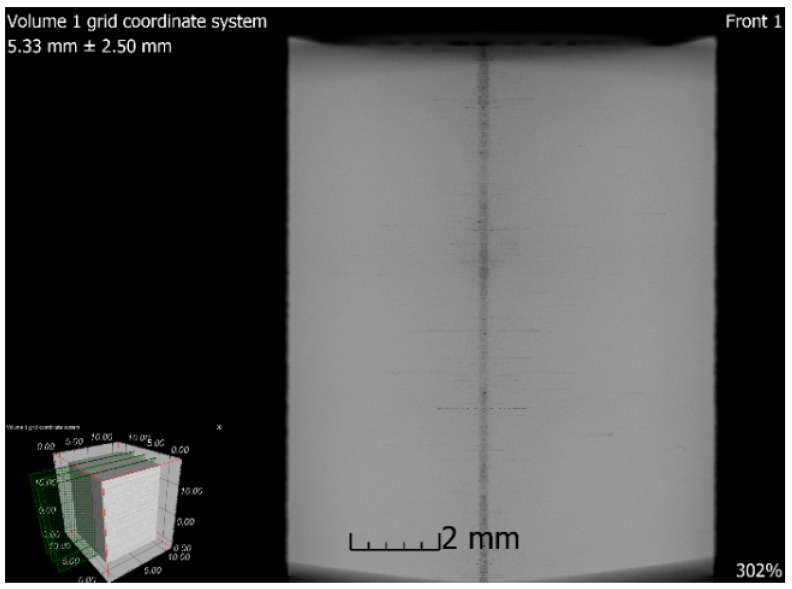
Tomographic CT sections of sample 5 after SLM + HIP_2 (XZ plane).
